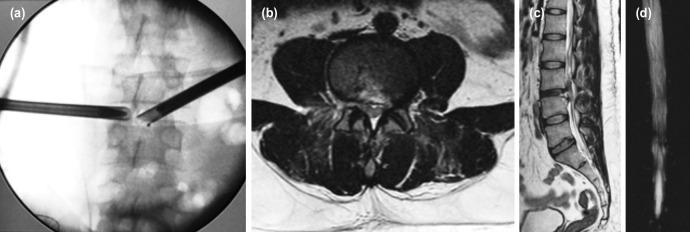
(a) “Inside out” Bi-lateral Bi-portal approach taken for surgery. (b,c) Post-operative T 2 axial and saggital MRI showing complete decompression. (d) MR myelogram shows blockage cleared and the patient recovered within one day.
